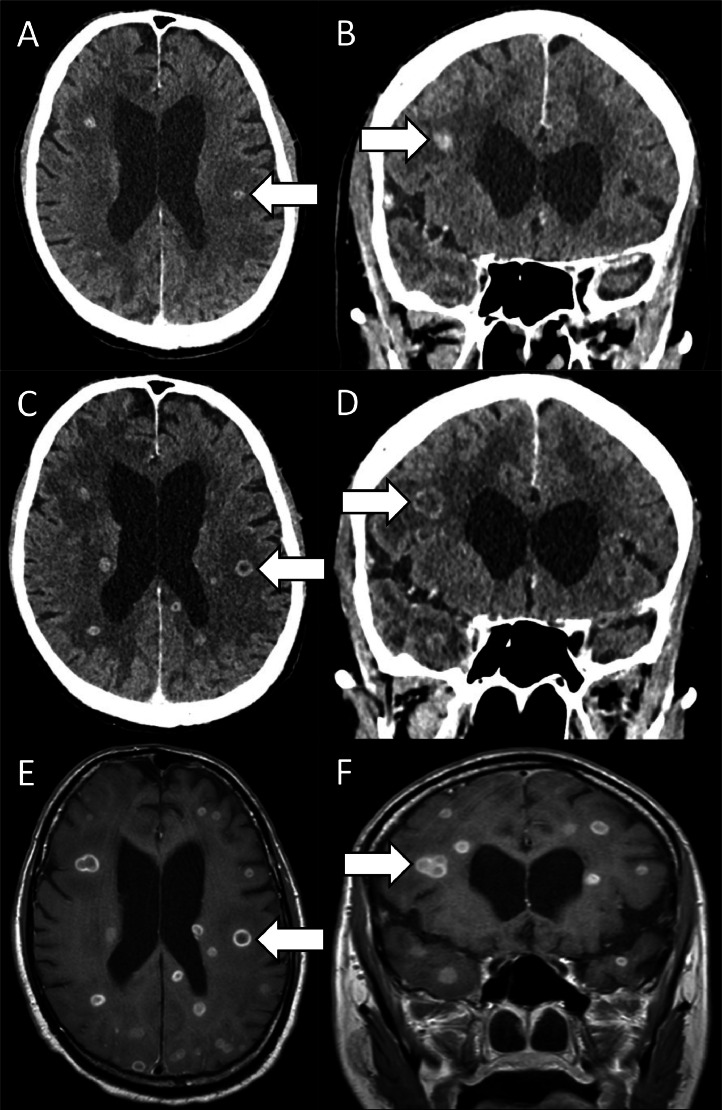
A contrast-enhanced CT and MRI of the brain at onset of neurological symptoms.
